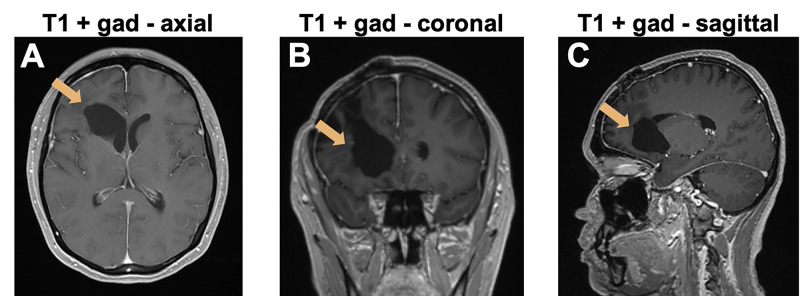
Delayed imaging two years after surgery A, B, C: T1-weighted MRI with gadolinium enhancement 25 months later shows no tumor recurrence. The arrows indicate the resection cavity.
